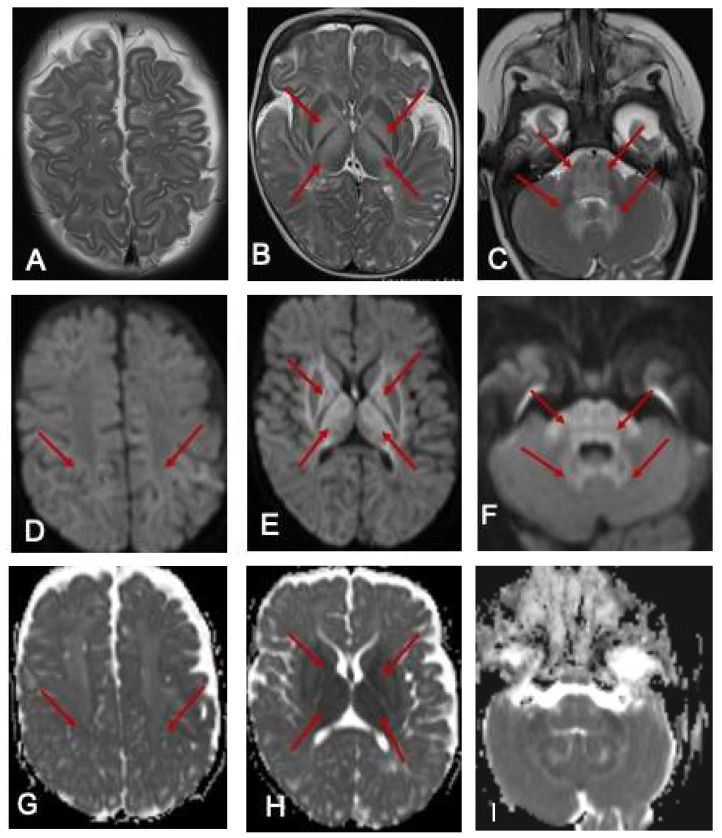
MRI of the brain at 7 months. The red arrows show the hyperintense signal changes. T2-weighted axial images of an MRI scan of the brain at 7 months of age (A–C) show hyperintense signal changes in the white matter (A), globus pallidus (B), and thalamus (B); pons, cerebellar peduncle, and dentate nucleus (C arrows). The corresponding areas show restricted diffusion in DWI Images (D–F) and ADC maps (G–I).
